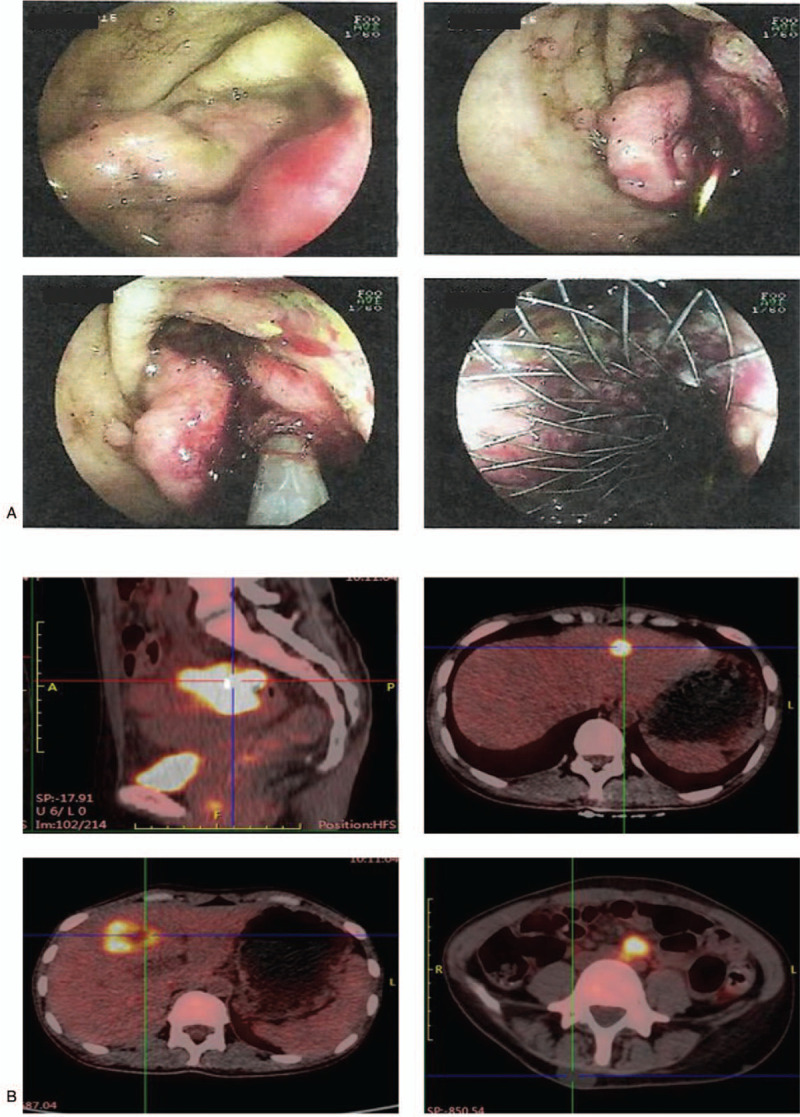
Gastroscopy revealed numerous sessile polyps of 0.2 to 0.5 cm extending from the gastric cardia to duodenum (A) and positron emission tomography-computed tomography (PET-CT) revealing high signaling in the recto-sigmoid junction, 2 high signals in the liver S3 and S4, and swelling retroperitoneal lymph nodes (B).
